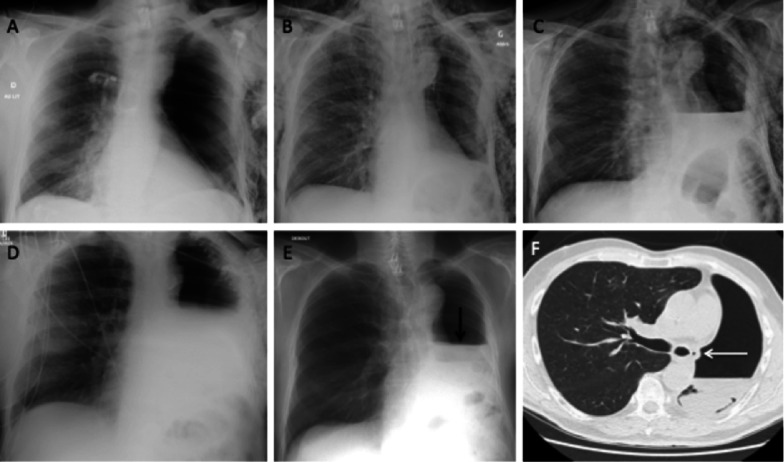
Bronchopleural fistula. A–D CXR 1 day, 3 days, 7 days and 10 days after surgery, with progressive elevation of the air–fluid level. E Febrile acute respiratory distress at day 28, CXR showing a decrease in the air–fluid level (black arrow). F Axial CT image (parenchymal window) at day 28 confirming that the air–fluid level is too low at this stage and showing an air bubble in contact with the bronchial stump (white arrow)Two major complications can occur consisting of pleural empyema and flooding of the contralateral lung with residual fluid from the lobectomy pouch leading to alveolar filling and ARDS.B.
